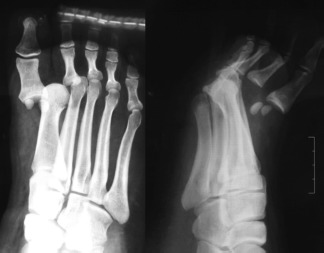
Preoperative radiographs AP and lateral views.
